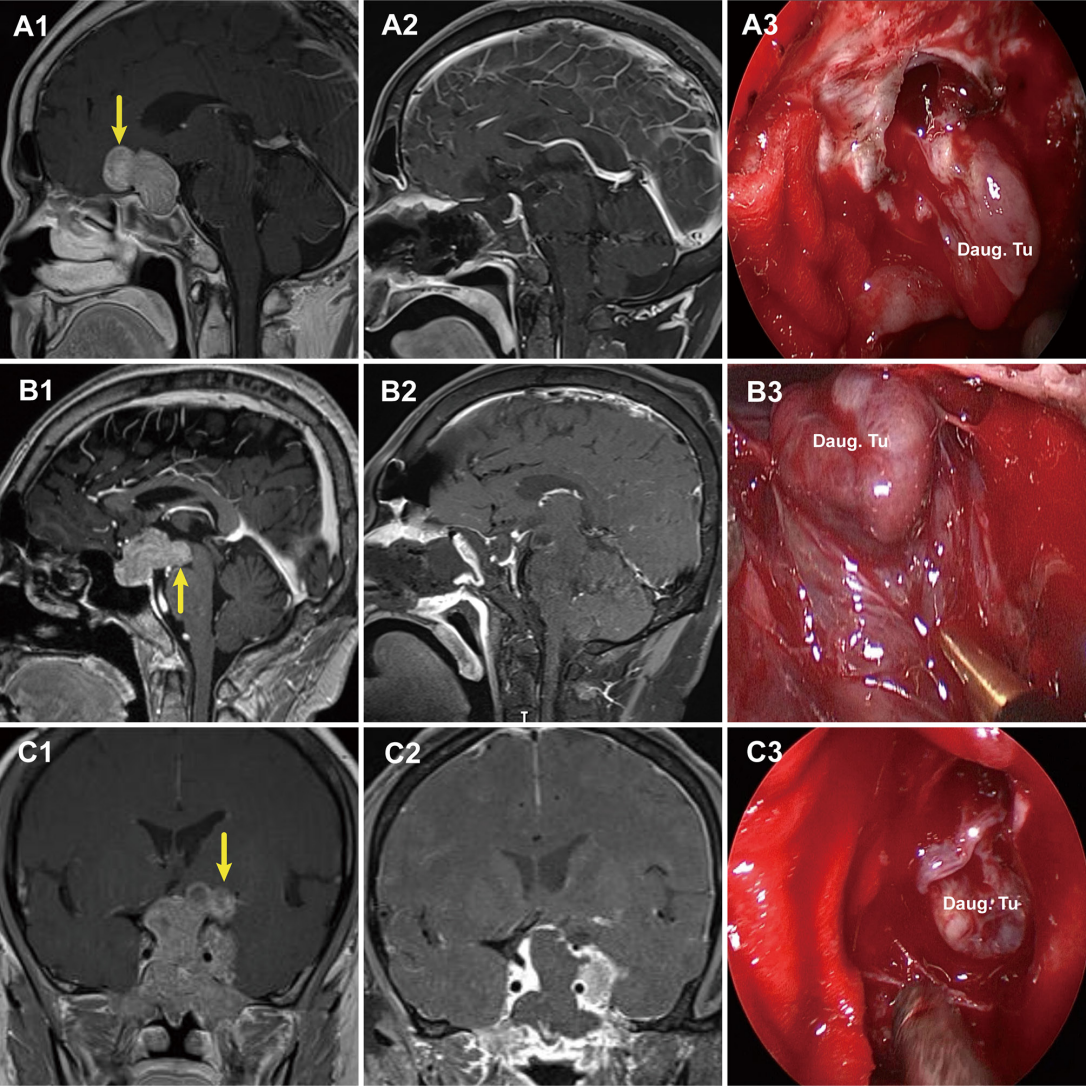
Three cases with grade 1b PAs that underwent endoscopic endonasal surgery. (A1, B1, C1) Preoperative, sagittal post-gadolinium MRIs showing 3 grade 1b tumors with daughter tumor (yellow arrow) extending to the anterior skull base (A1), the interpeduncular fossa (B1), and the suprasellar lateral (C1). (A2, B2, C2) Postoperative MRI demonstrating gross total resection of suprasellar part of tumor was achieved. (A3, B3, C3) Endoscopic views showing the intact wall of daughter tumor. Daug. Tu, daughter tumor; DS, diaphragm sellae.
